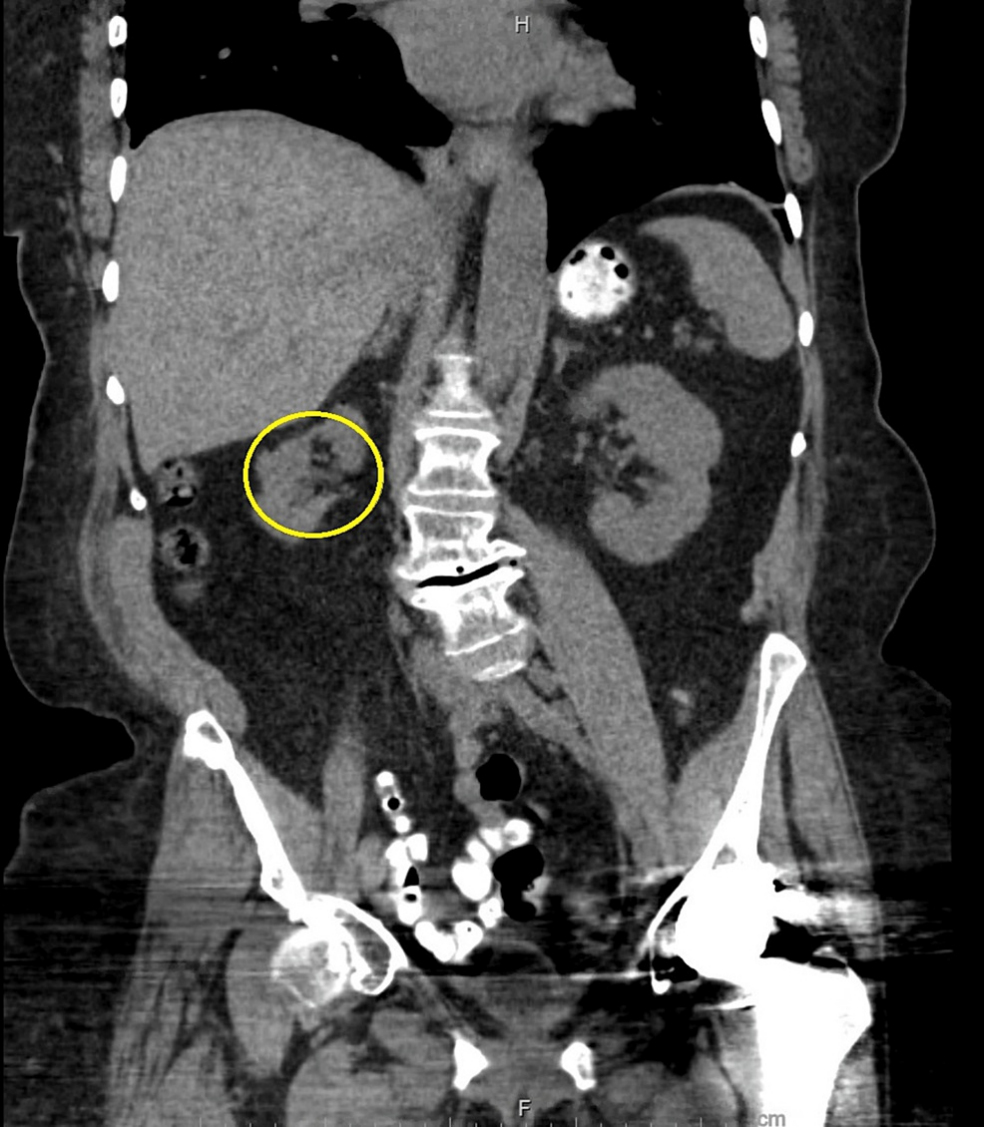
CT scan of the abdomen. *Yellow circle*: Right kidney is atrophic with chronic and irregular thickening of the parenchyma noted. CT, computed tomography
